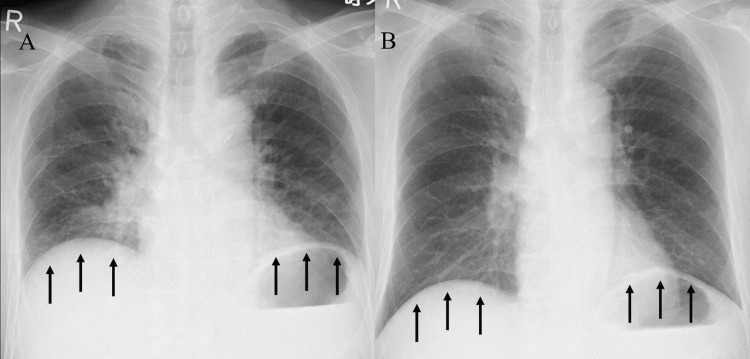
Follow-up chest radiographs showing improved diaphragmatic movement. Follow-up chest radiographs demonstrate a discernible change in diaphragm position between expiration (A) and inspiration (B), indicating improved diaphragmatic function. The black arrow points to the diaphragm, with improved movement during breathing.
